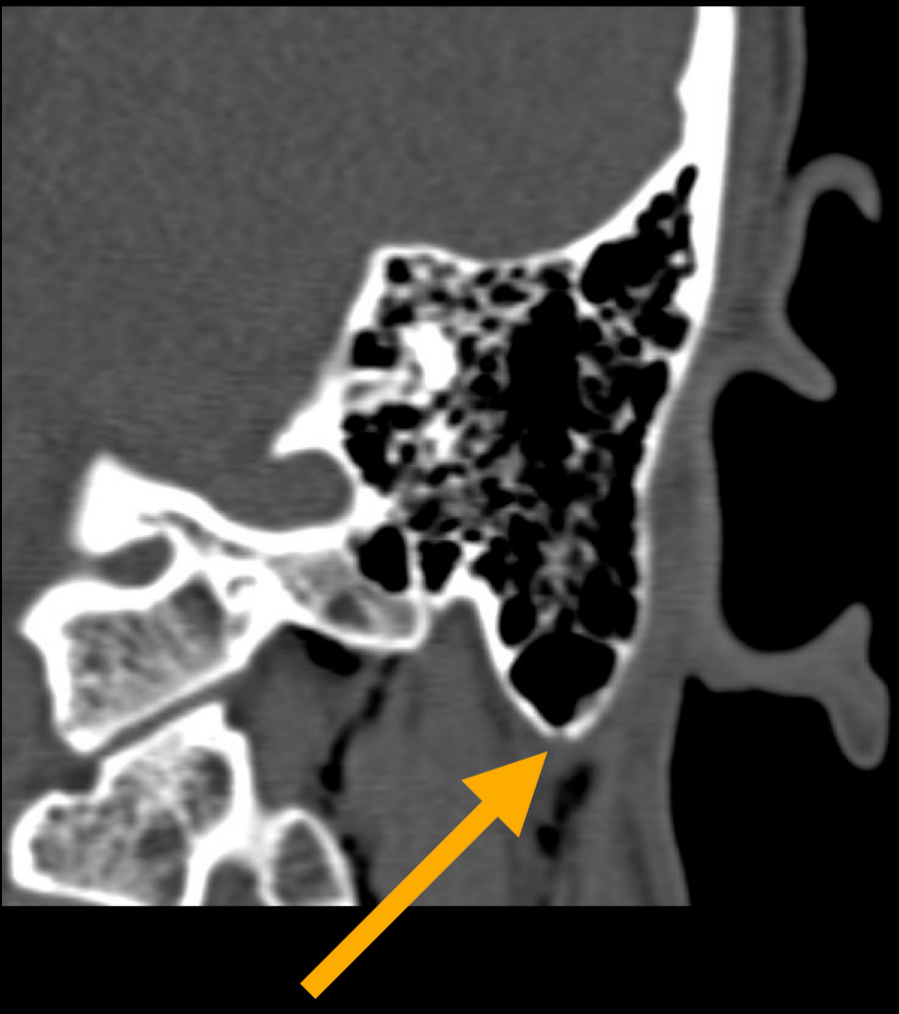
Coronal plane HRCT scan – Left side: caudal mastoid apex fracture (yellow arrow), with presence of SCE
